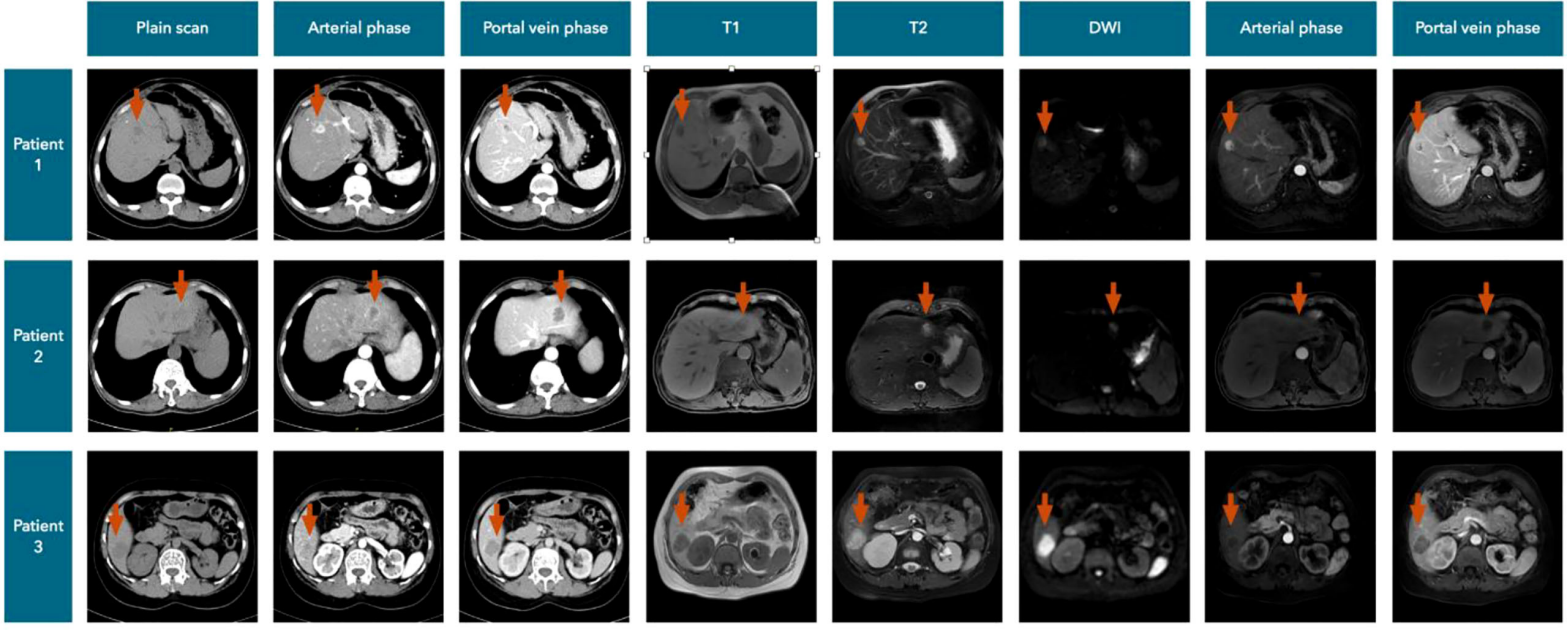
CT and MRI images of three patients.
